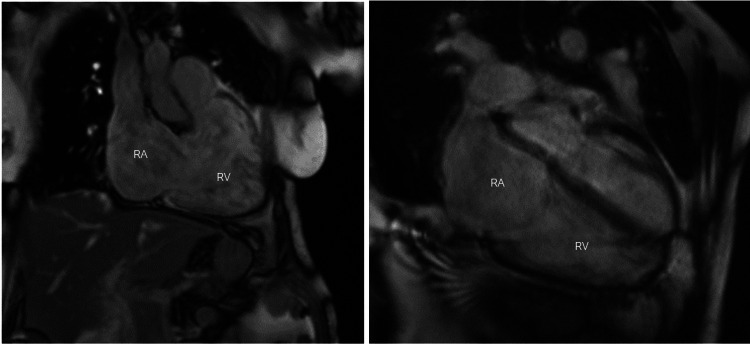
Cardiac magnetic resonance imaging Cardiac MRI showing dilated RA and RV. The RV is severely dilated in size with normal systolic function (RVEF = 54%). The anomalous left pulmonary venous drainage into the RA could not be visualized in this study. MRI, magnetic resonance imaging; RA, right atrium; RV, right ventricle; EF, ejection fraction.
